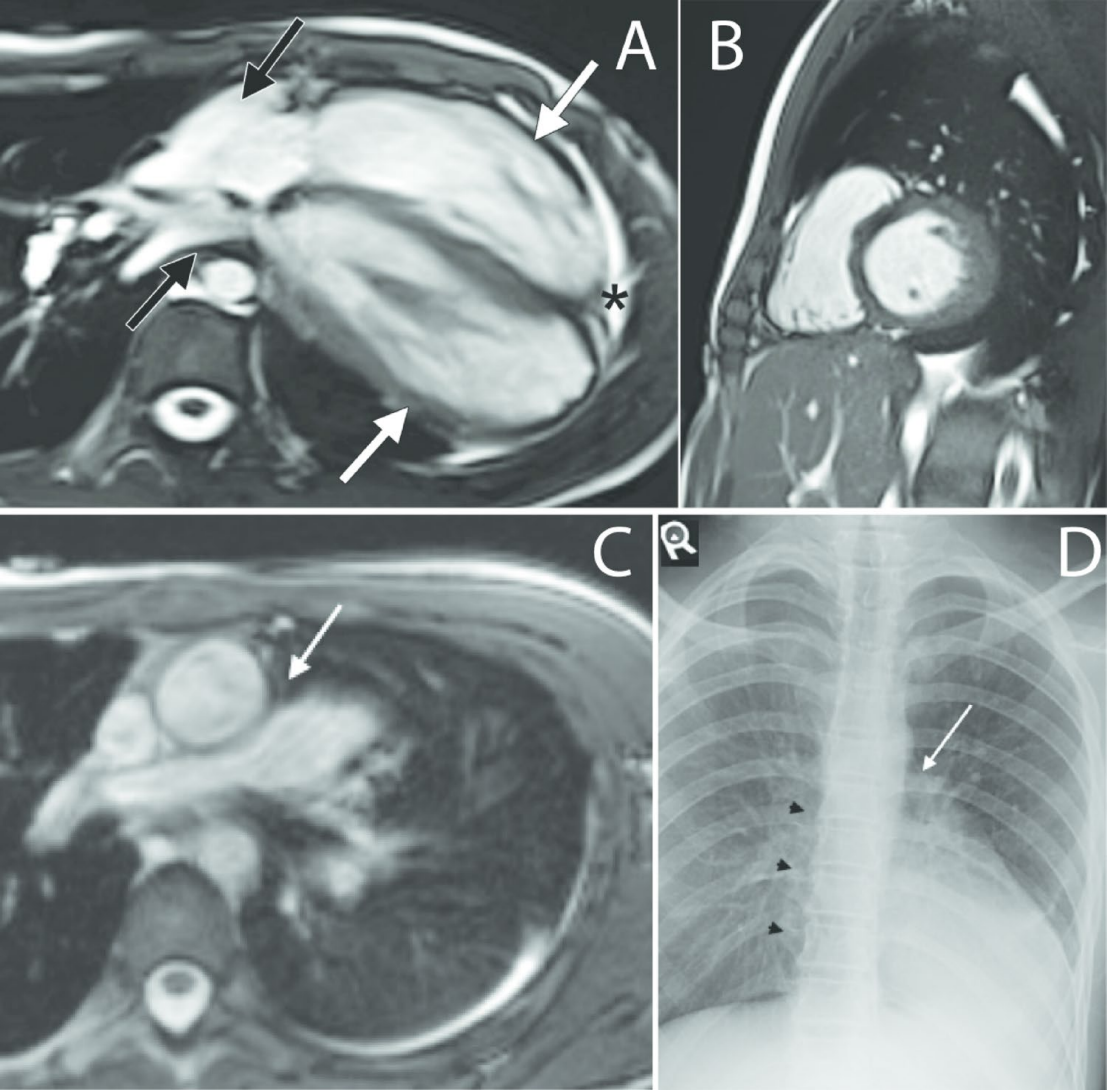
(A) Cardiac MRI SSFP cine image in four-chamber view shows elongated appearance of both atria (black arrows) and relatively dilated ventricles (white arrows) giving tear drop morphology. The pericardium is not seen in the fat surrounding the heart (*). (B) Cardiac MRI Steady State Free Precession (SSFP) cine image in short-axis view shows a portion of the left lung (black arrow) insinuate below the inferior wall of the left ventricle (white arrow) during the cardiac cycle. (C) Cardiac MRI SSFP cine image in axial view showing interposition of lung tissue between the ascending aorta and main pulmonary artery (white arrow). (D) The chest X-ray demonstrates straightening and elongation of the left cardiac border, loss of right cardiac border (black arrow heads), and interposition of pulmonary tissue between the aortic knob and main pulmonary artery (white arrow)
